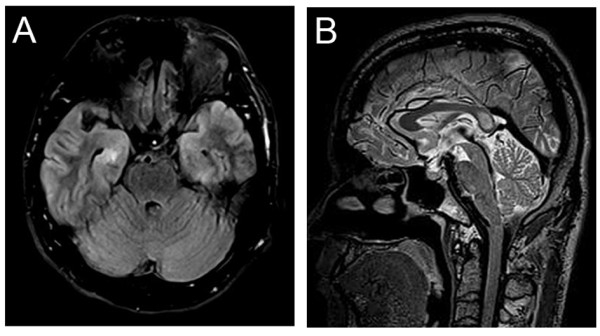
MRI scans of HE patients. (A and B) Patient No.4 with memory impairment. The splenium corporis callosi and bilaterial hippocampal abnormalities are believed to be responsible for the memory impairment.
